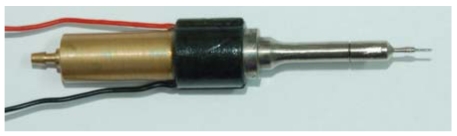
A picture of the ultrasonic probe
